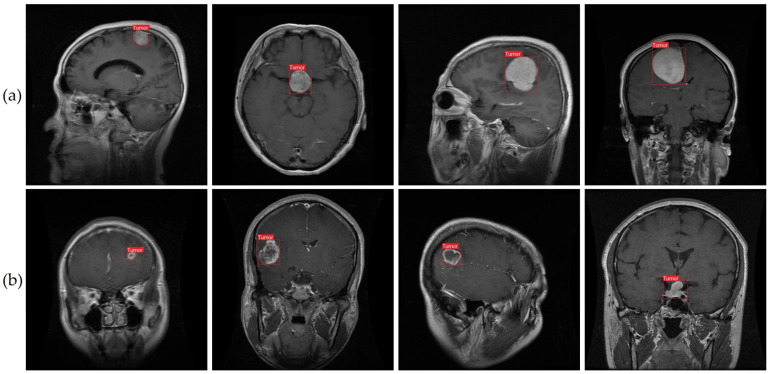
Visual representation of brain tumor detection from MRI images. (a) Big size of brain tumor detection, (b) Smaller size of brain tumor detection.
